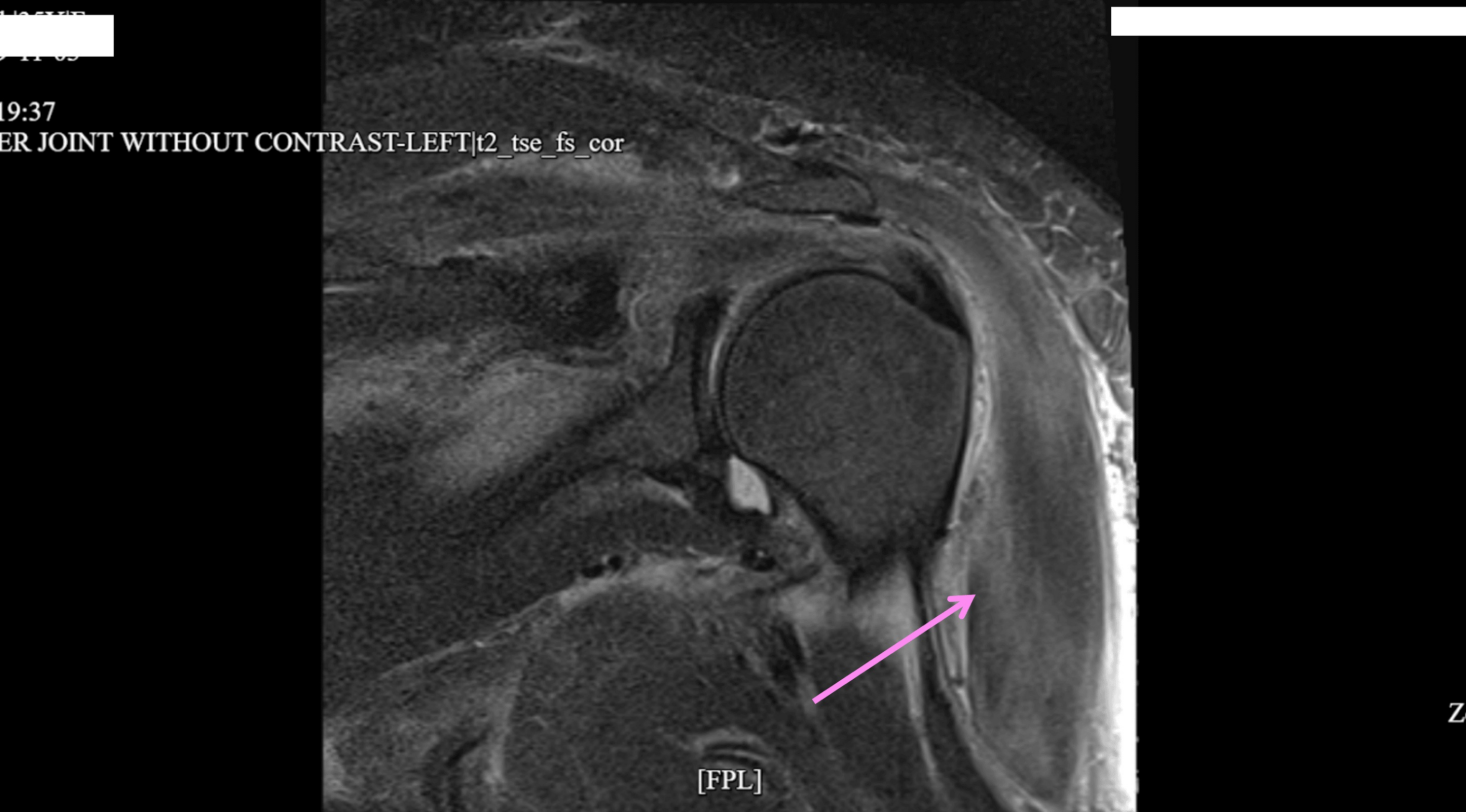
Magnetic resonance imaging without contrast showing diffuse cellulitis and myositis of the left deltoid.
